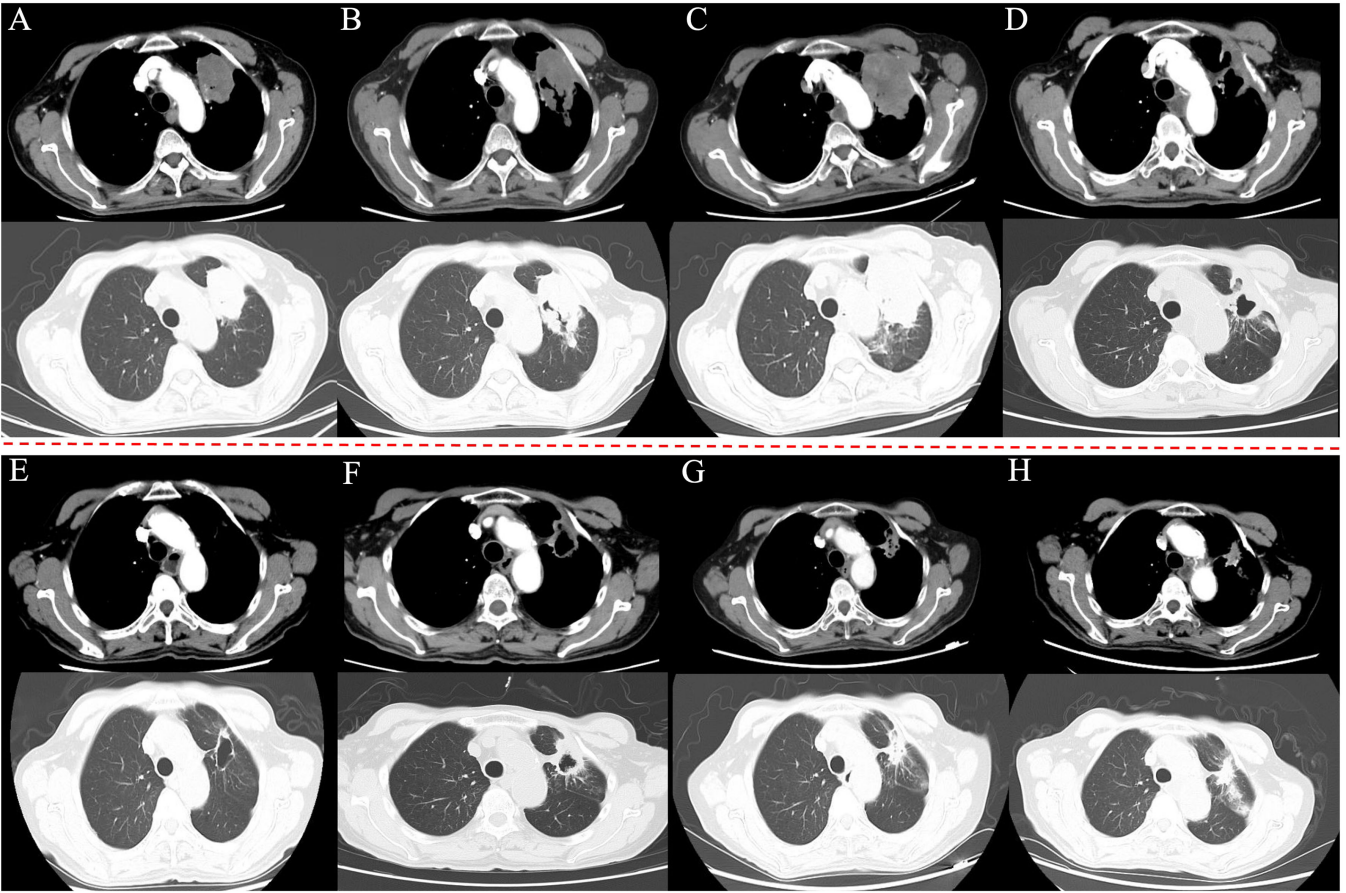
Chest CT scan of the patient during the treatment process at both the mediastinum window (above) and the lung window (below). (A) Initial imaging revealed a 5.7×4.2 cm mass of soft tissue-like density in the left upper lobe of the left lung. (B) After 2 months of crizotinib, the lung lesion (5.6×4.4 cm) assessed as stable disease. (C) After 7 months of crizotinib, the lung lesion size increased to 6.8×4.8 cm. (D) After two cycles of camrelizumab, the lung lesion size reduced to 3.6×3.0 cm. (E) After 18 months of camrelizumab maintenance, almost no tumors left in the mediastinal window. (F) After 20 months of camrelizumab maintenance, the lung lesion size increased to 4.2×3.0 cm. (G) After 2 months of voriconazole, the lung lesion size reduced to 3.0×2.4 cm. (H) At 41 months from WBRT initiation (with subsequent camrelizumab), the lung lesion (3.3×2.2 cm) assessed as stable disease (latest image).
